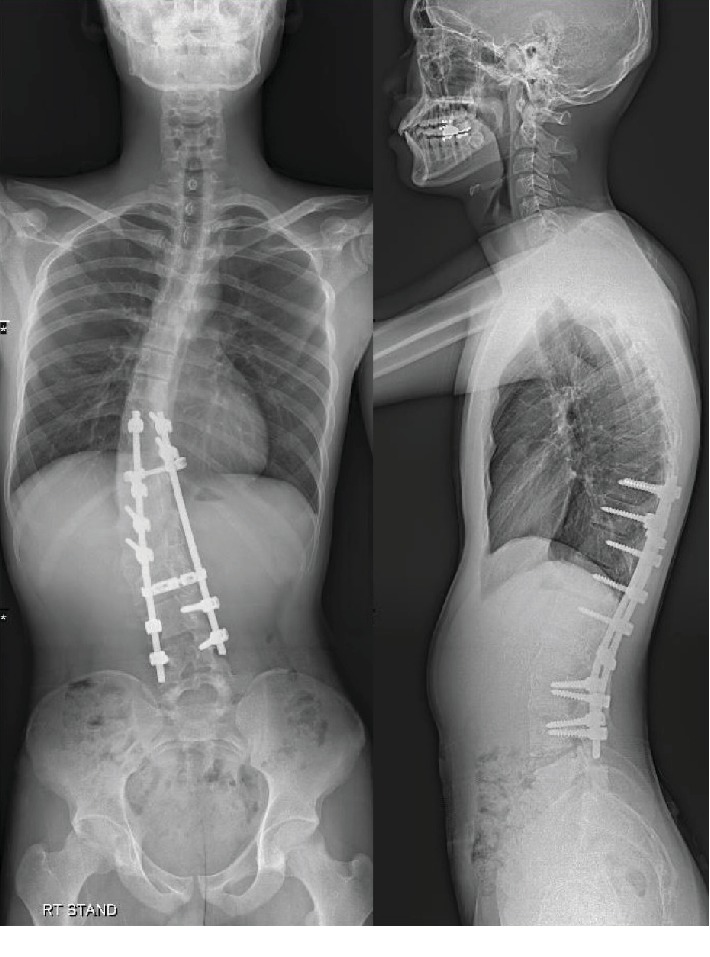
Whole spine plain radiograph at the follow-up visit to outpatient clinic, at March 11^th^, 2019, 3 years and 4 months are passed since the surgery.
